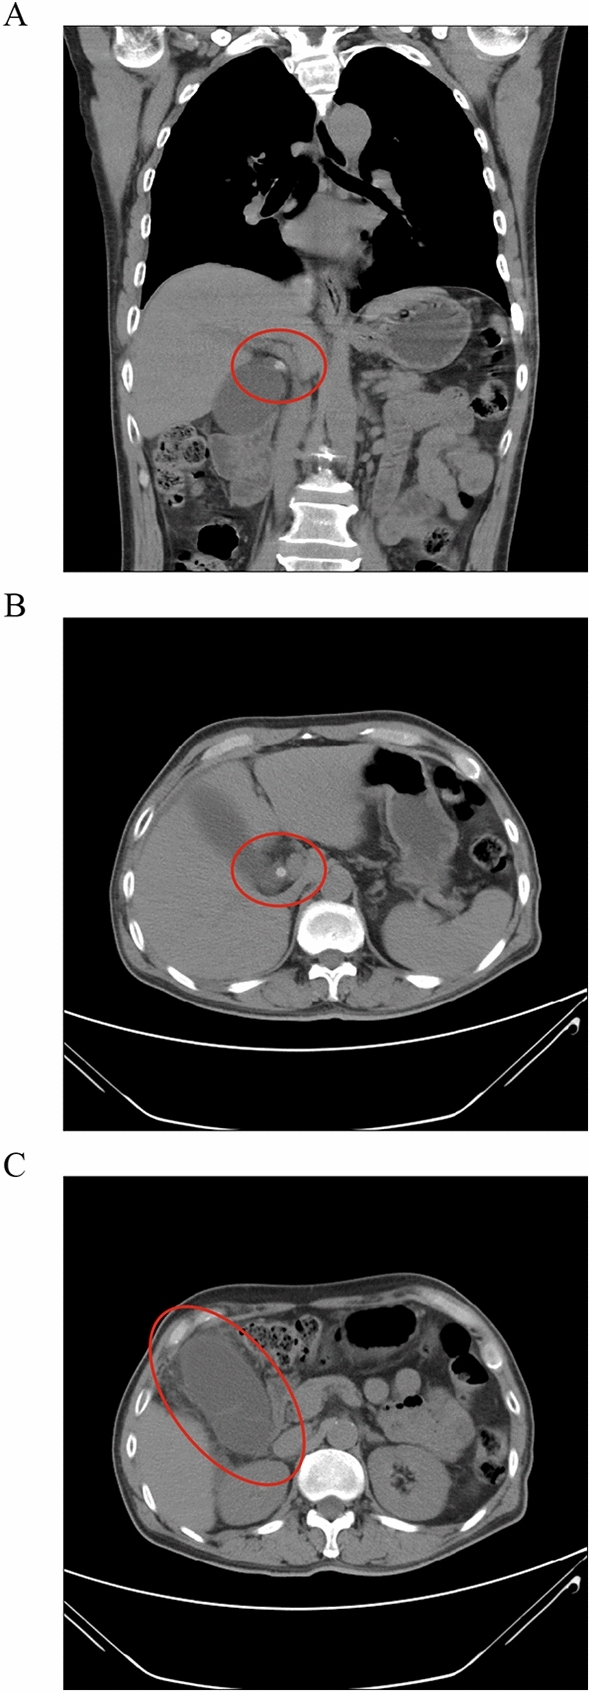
Interpretation of specific clinical features. (A) CT scan showed obstruction of stones in the cystic duct (coronal view); (B) CT scan showed obstruction of stones in the cystic duct (axial view); (C) CT scan showed inflammatory reaction around the gallbladder.
